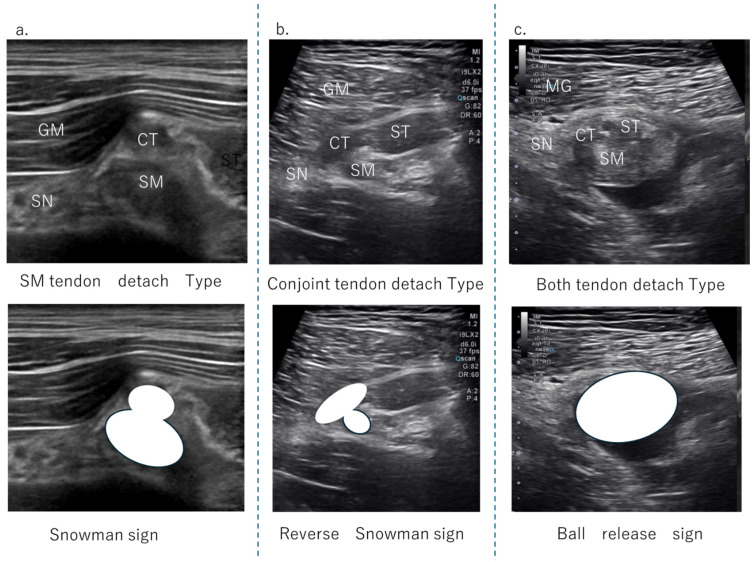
The US findings of the tendon detachment. (a) This is a case of SM detachment from the attachment point. Compared to CT, the SMT that exists in the deeper layers regresses, and the volume in this area often increases, resulting in an image like a ‘snowman sign’. (b) If there is a tear or damage to the CT, the surface layer will become a larger oval, presenting a ‘reverse snowman sign’ appearance. (c) When both the CT and SMT are torn apart as a whole, they are depicted as a single mass, or ‘ball release sign’. This expresses the appearance of the attachment area separating from the ischial tuberosity as a single mass, like a ball.
